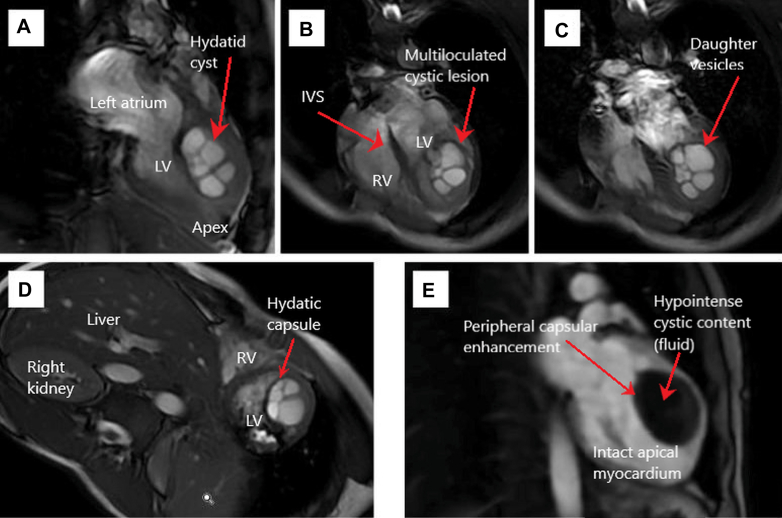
Cardiac Magnetic Resonance Imaging Cine-SSFP sequences in (A) sagittal and (B to D) axial planes show a multiloculated cystic lesion in the apex of the LV, containing multiple daughter vesicles—an imaging feature highly suggestive of an active hydatid cyst. (E) T1-weighted sequence with late gadolinium enhancement in the sagittal view demonstrates a unilocular cystic lesion with homogeneously hypointense content (consistent with fluid) and peripheral capsular enhancement, located in the apical region of the LV. This sequence allows assessment of capsular integrity and helps rule out myocardial infiltration or rupture. SSFP = steady-state free precession; other abbreviations as in Figure 2.
